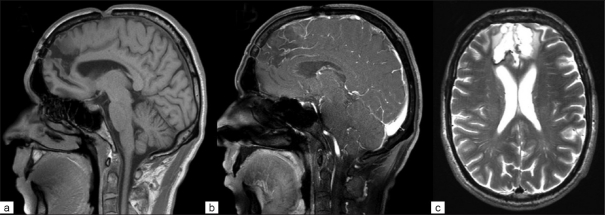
Postoperative magnetic resonance imaging showing no signs of tumor growth; (a) sagittal T1-weighted scan; (b) sagittal gadolinium-enhanced T1-weighted scan; (c) axial T2-weighted scan
